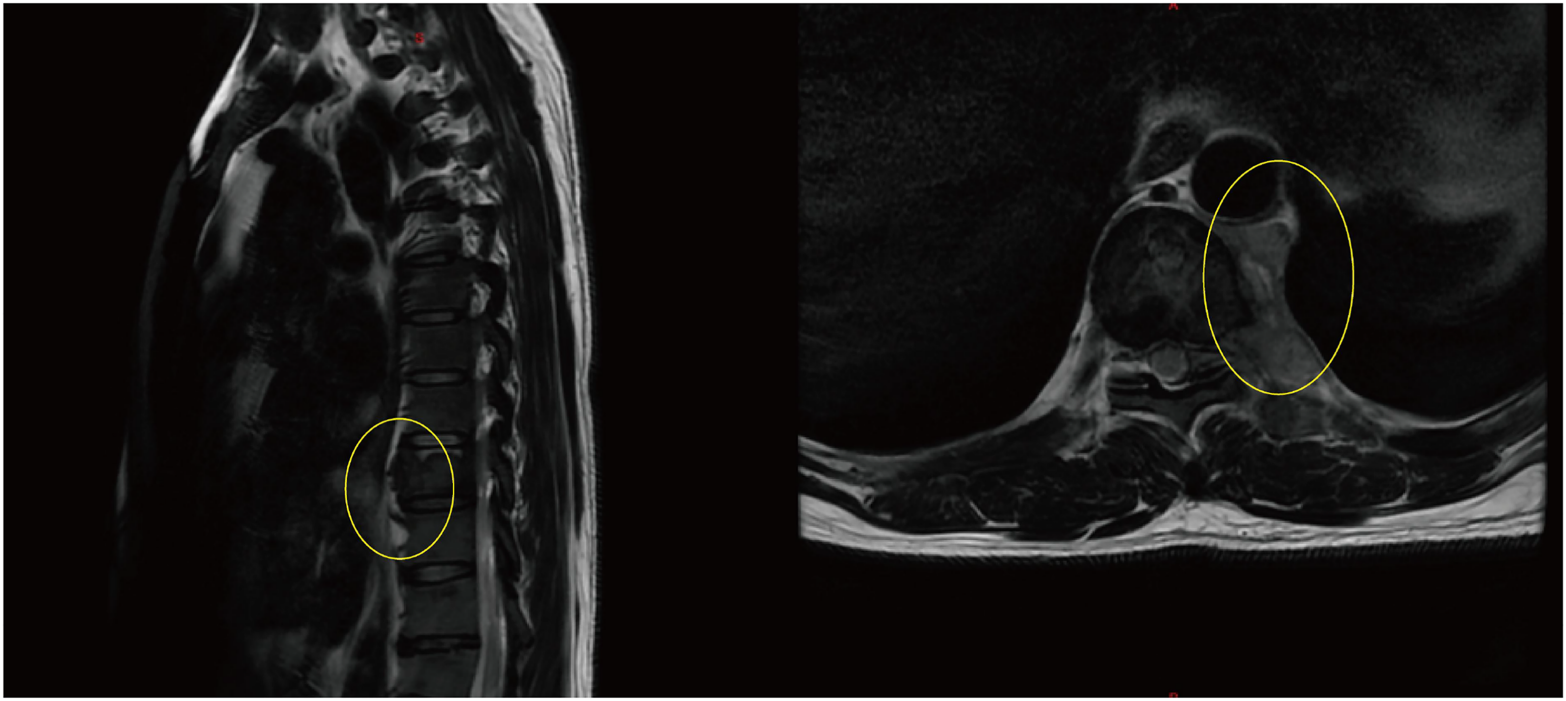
Thoracic spine MRI showing destruction of the T9 vertebral body with adjacent paravertebral soft- tissue mass (The yellow circles indicate the location of the myeloid sarcoma.).
